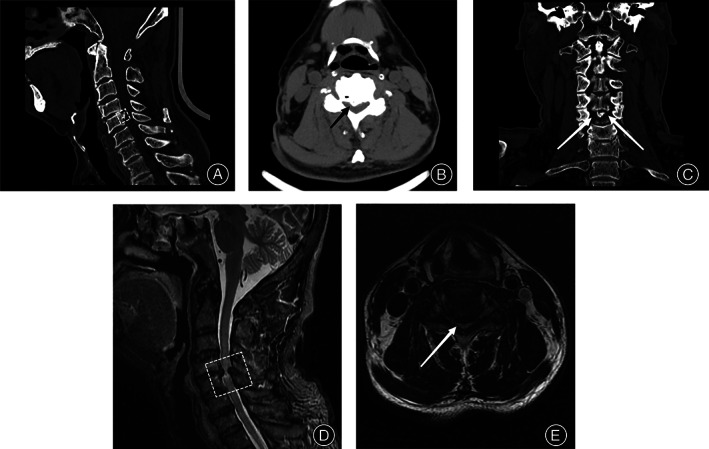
A 72‐year‐old man presented with a complaint of neck pain accompanied by numbness and weakness of the extremities. The muscle strength of each limb was Grade 3, the muscle tension of both lower limbs was slightly increased, and the knee tendon and Achilles tendon reflexes were hyperactive. The following are the preoperative radiology data: (A) The sagittal plane of CT showed that the ossification originated in the middle of the C5 vertebral body, with a width of 4.3 mm and a height of 6.1 mm. (B) The axial plane of CT showed that the ossification was 8.9 mm long and 4.3 mm wide, and the posterior protrusion compressed the spinal canal. (C) The coronal plane of CT showed that the ossification was located in the middle of the vertebral body of C5. (D) Sagittal MRI showed compression of the spinal cord at the C4/5 level, with hyperintensity and suggestive degeneration (the dashed boxes indicate the compressed segments). (E) The anterior and posterior diameter of the spinal canal was 8.1 mm and the OR was 0.6, indicating a high degree of compression
